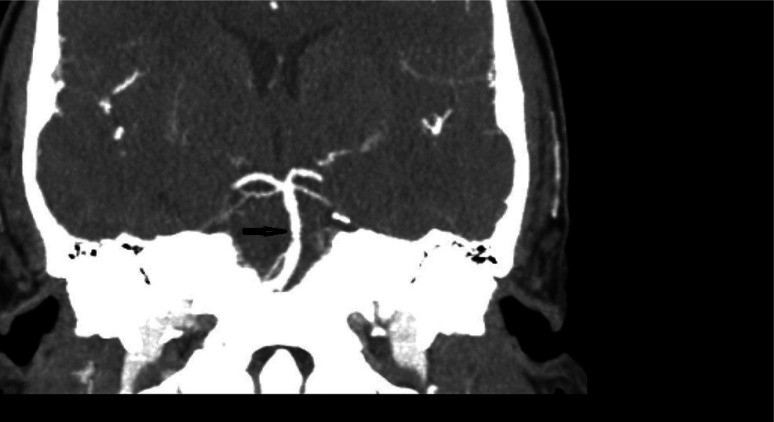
CTA coronal section showing midbasilar stenosis due to dissection.
